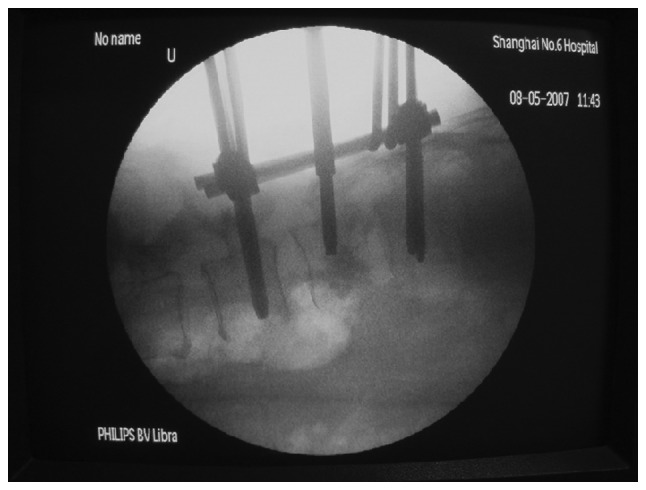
Intraoperative bone implant status examined by C-arm X-ray.
